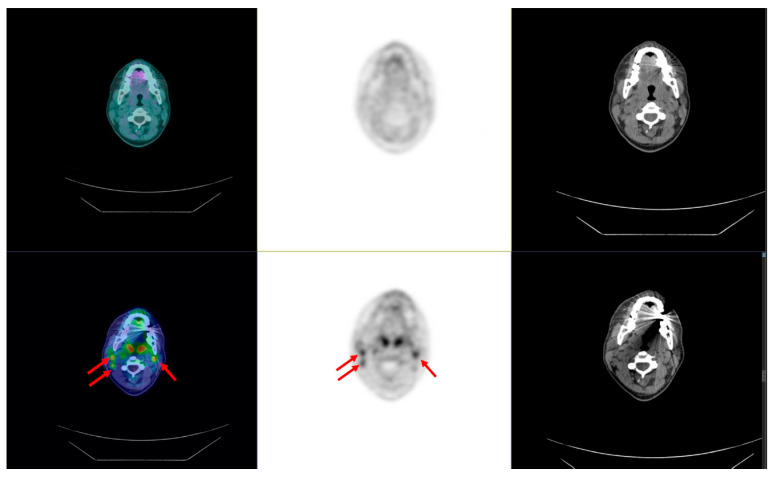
Bifocal and bilateral medullary thyroid carcinoma. Preoperative PET/CT: arrows showing bilateral lymph node metastases negative at [^18^F]FDOPA (top) and positive at [^18^F]F-SiFAlinTATE (bottom).
